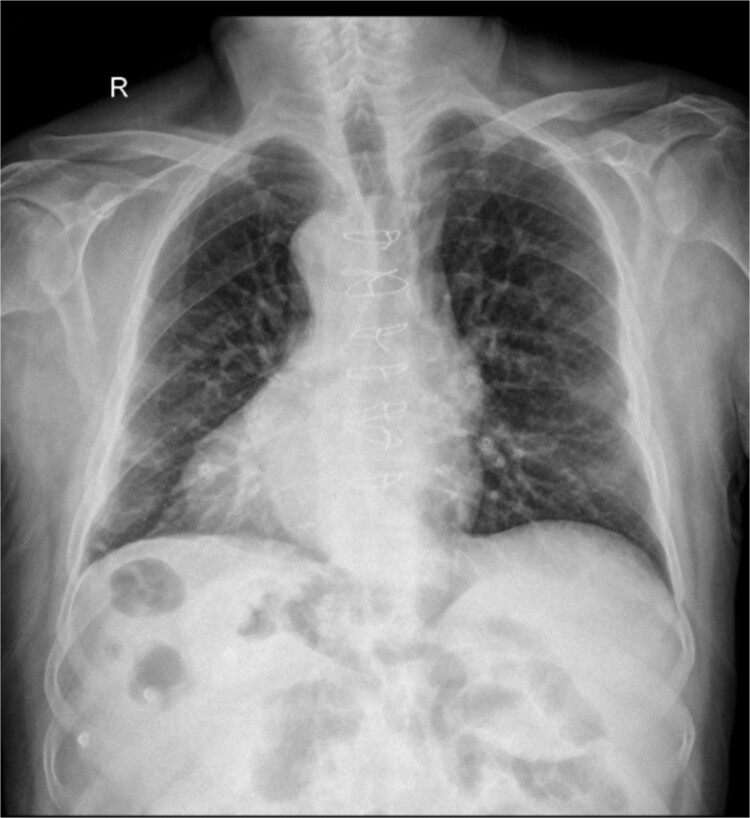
Chest X-ray showing dextrocardia.
